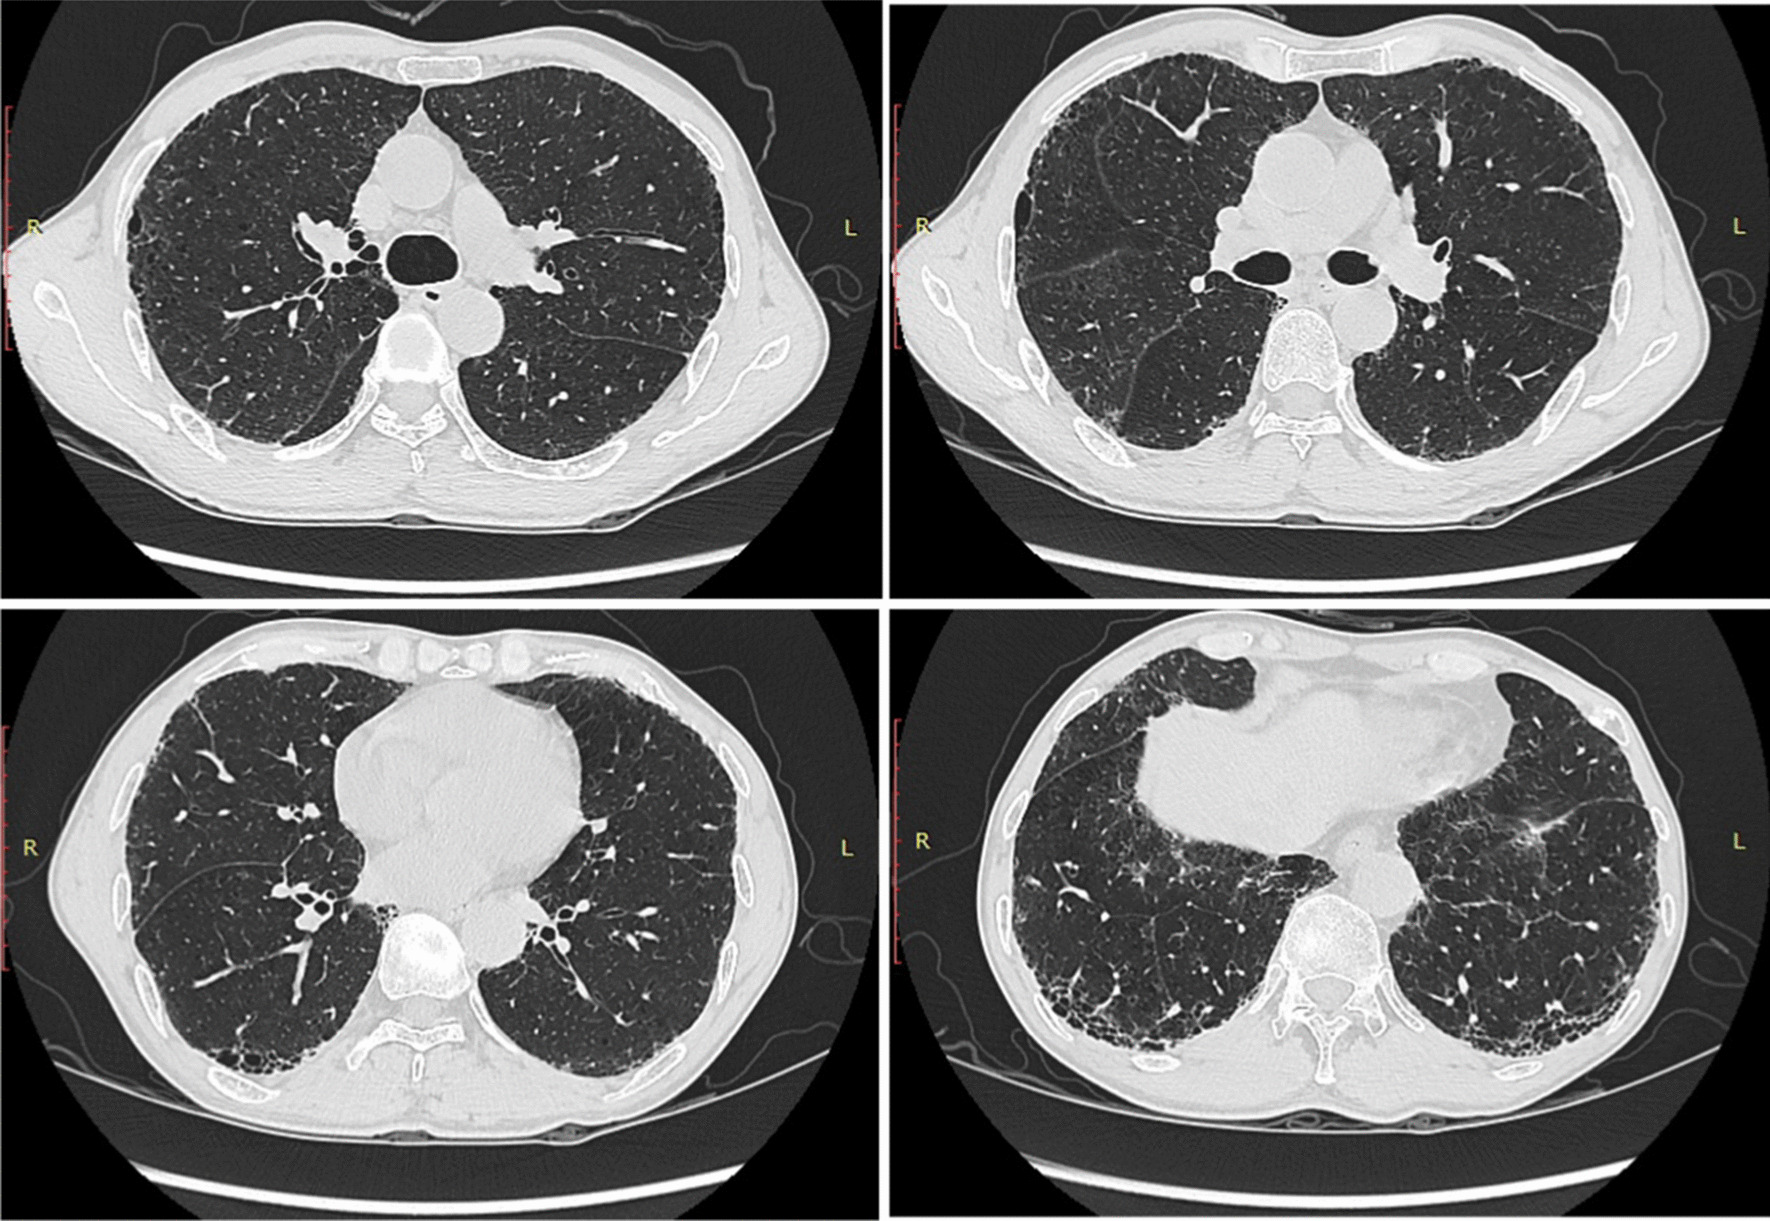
Initial chest HRCT scan performed on July 19th, 2019. Photos indicate interstitial lung inflammation with imaging features of NSIP
